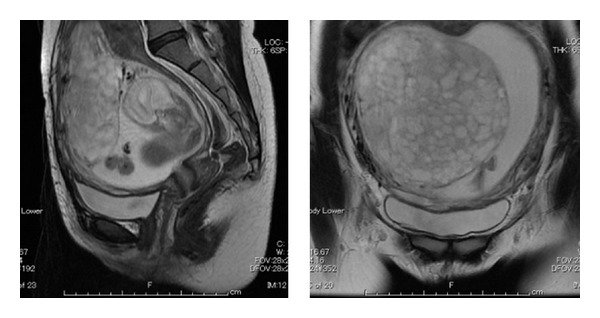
Magnetic resonance imaging showing a large multicystic placenta at 20th weeks of gestation. Normal part of the placenta was not clearly identified.
